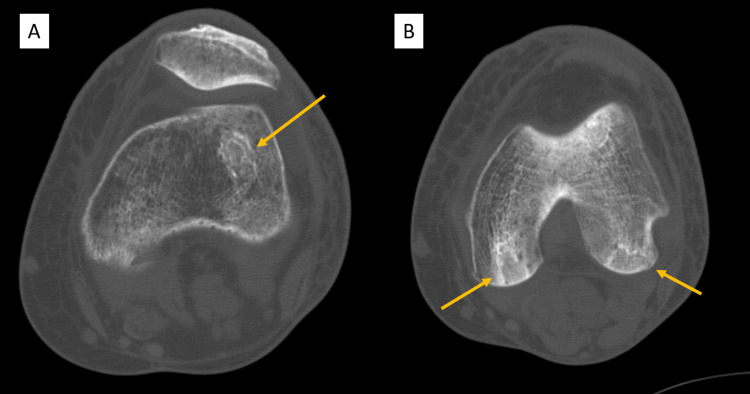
Left knee computed tomography scan without contrast depicting areas suspicious for osteonecrosis in the bilateral femoral condyle (yellow arrows).
